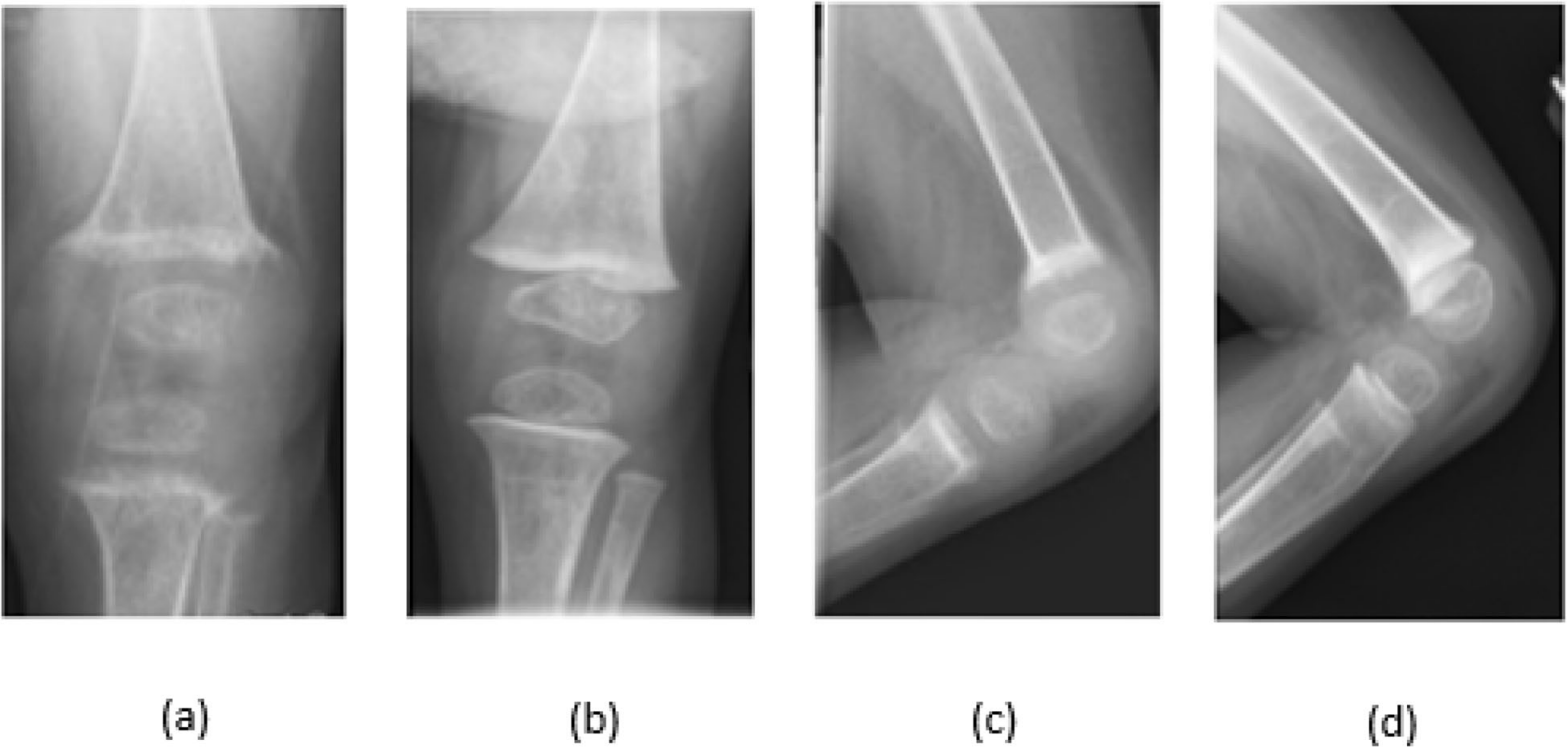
Twin* B knee radiographs. AP view (a) before treatment (b) after treatment. Lateral view (c) before treatment and (d) after treatment. a and c show diffuse osteopenia, fraying and widening of metaphyses. b and d demonstrate improvement in rickets
